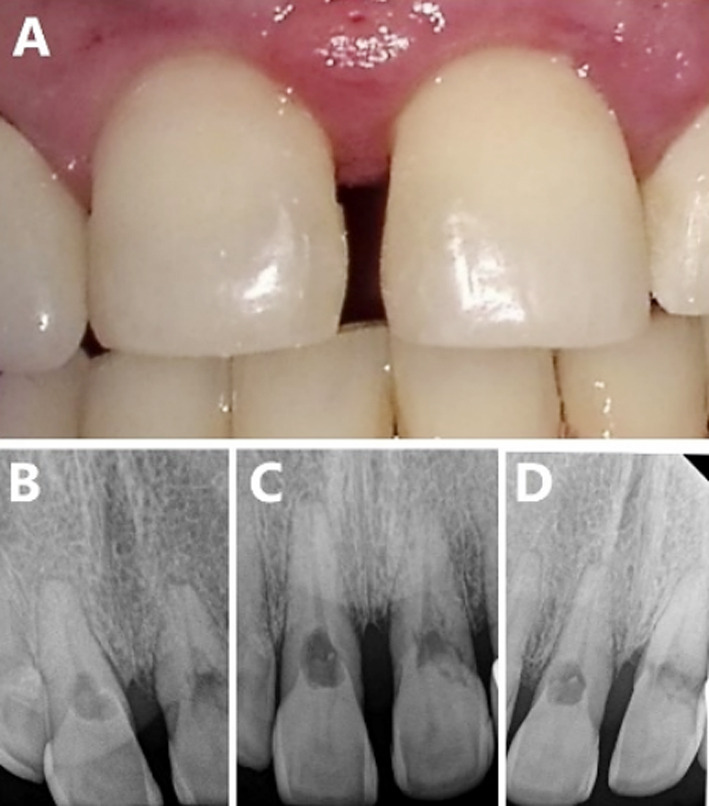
(A) Clinical appearance of Maxillary central incisors, Periapical Radiographs (PAR) (B) Mesially shifted PAR, (C) PAR, (D) Distally shifted PAR.
